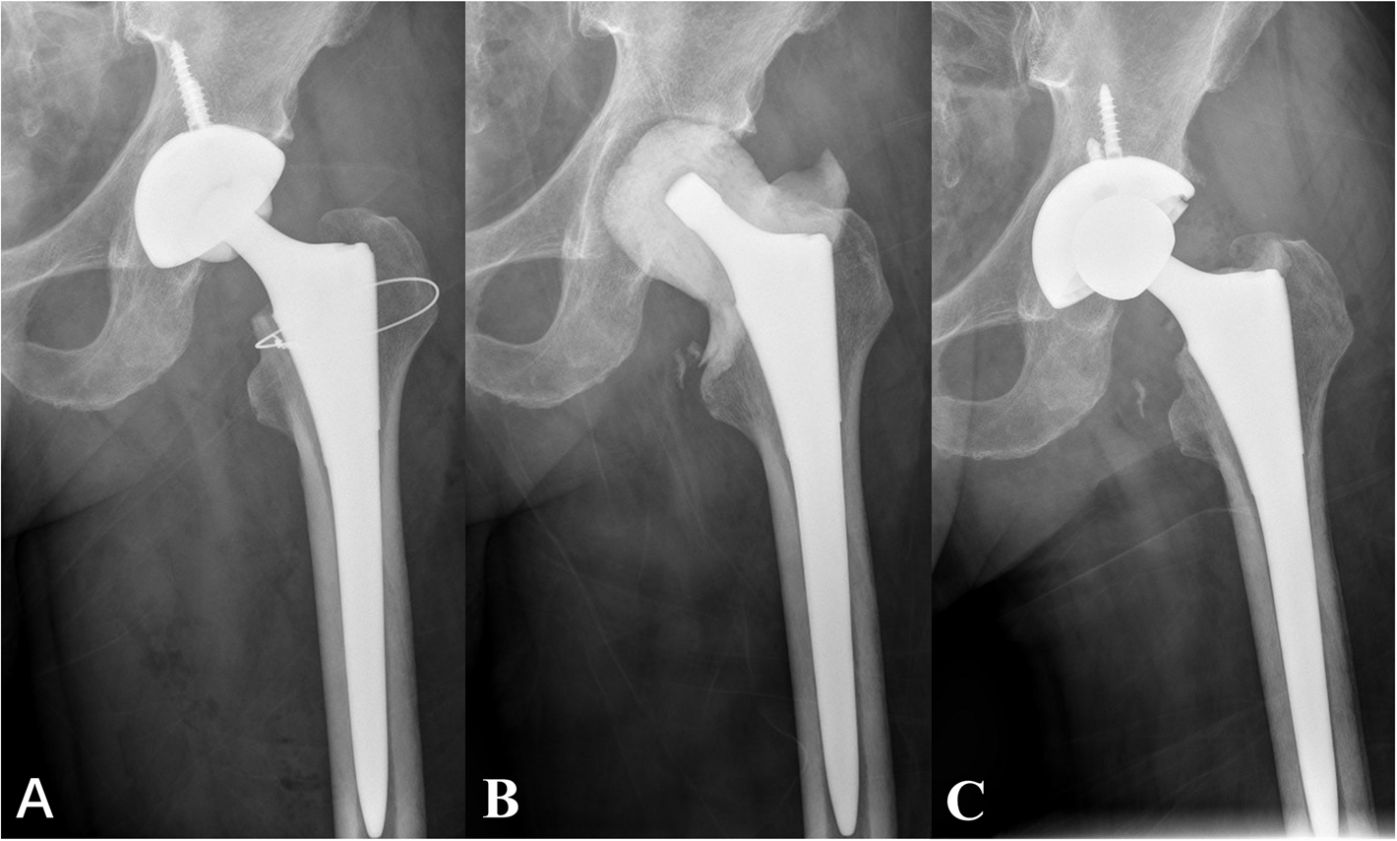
Left hip anteroposterior radiograph of patient 22. This patient underwent left THA 6 years previously. (A) The X-ray showed evidence of loosening of prosthesis but the femoral stem. (B) The acetabular cup was removed, and a cement spacer was inserted in the first-stage operation. (C) Until the 3-year follow-up, the patient was free of infection
